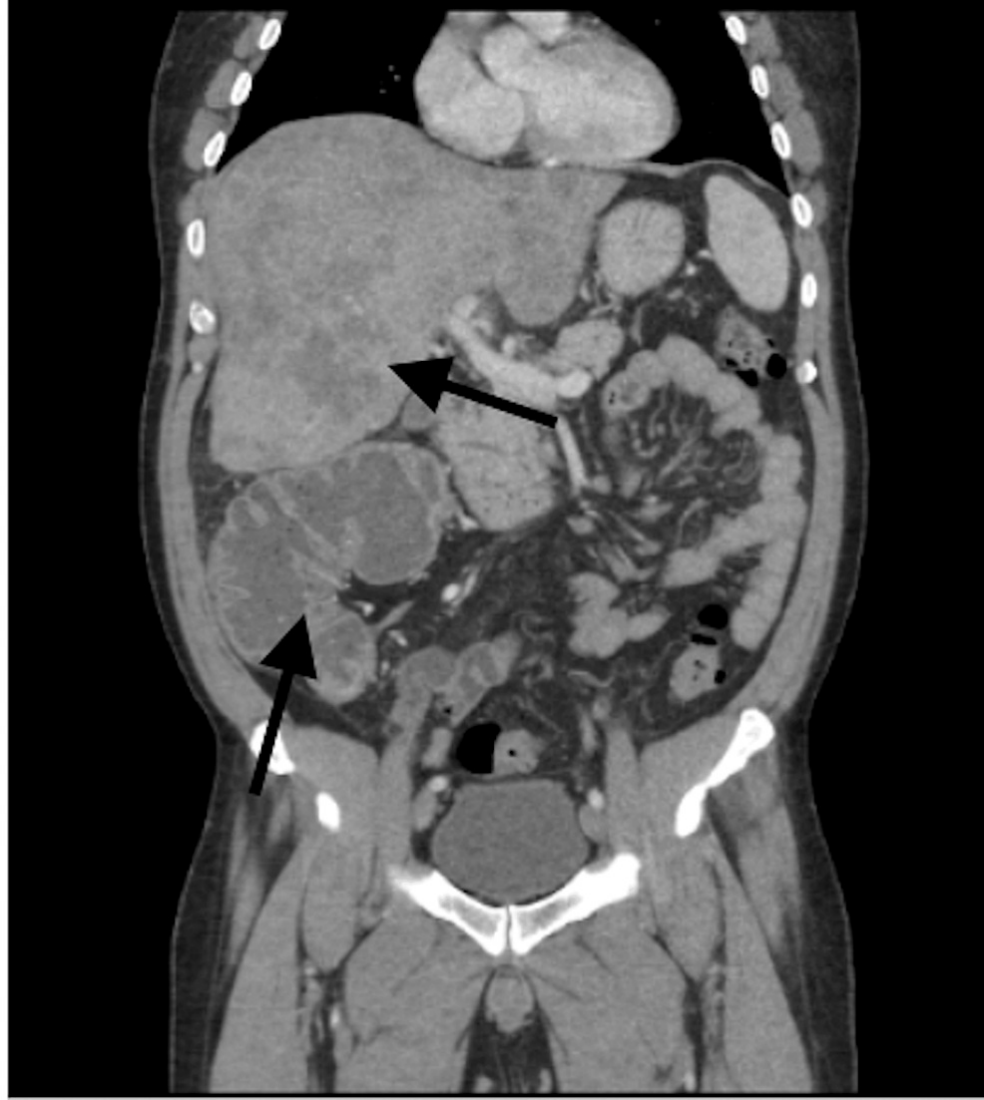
CT abdomen and pelvis with contrast, coronal view, demonstrating large bowel obstruction in the transverse colon and liver metastases.
